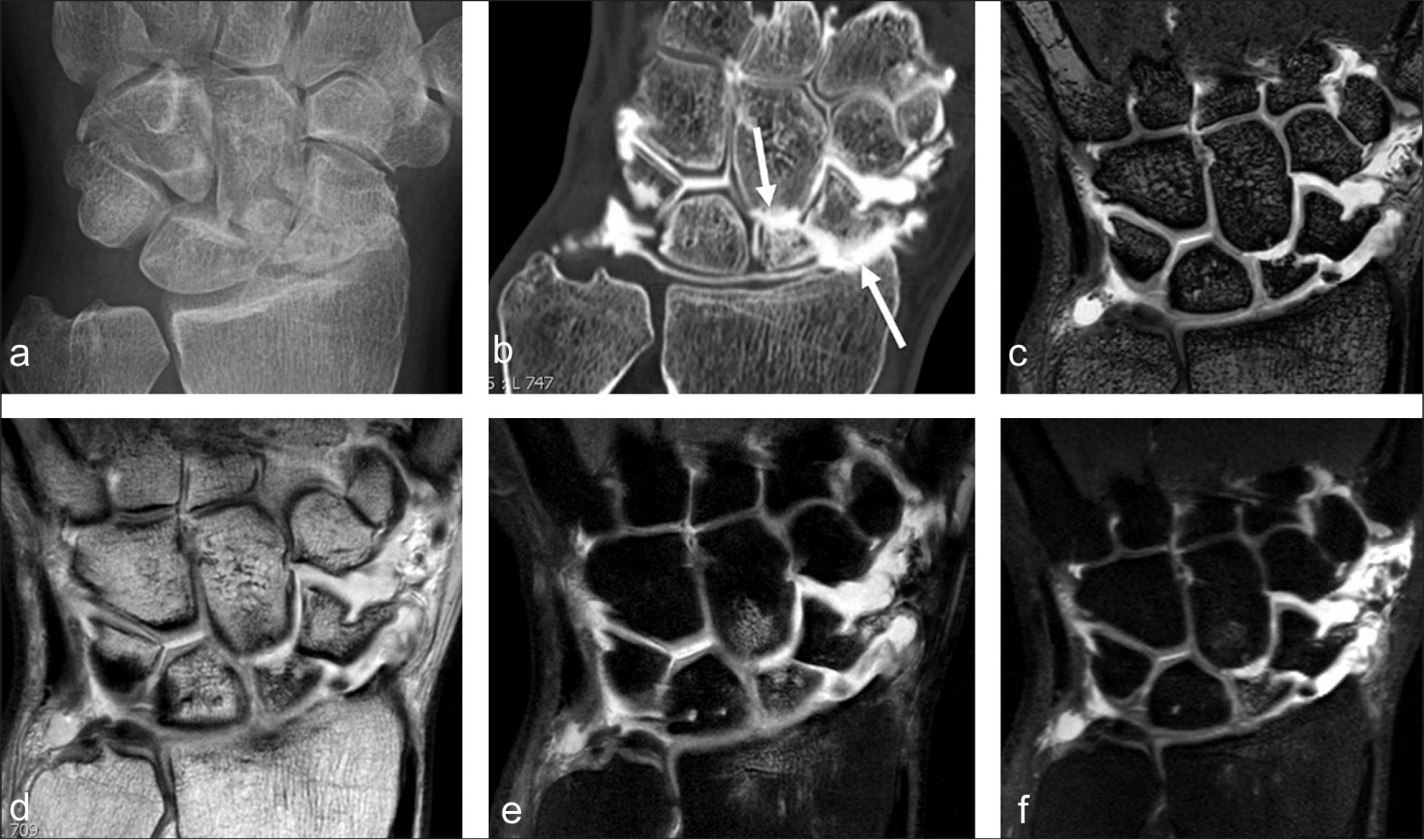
SNAC 2 wrist after scaphoid fracture with nonunion in a 41-year-old man. Secondary cartilage degeneration is first seen in the radioscaphoid compartment (b, arrow pointing up) and later in the scaphocapitate midcarpal joint space (b, arrow pointing down) on conventional radiograph and much better on CT-arthrography (b). MRI well demonstrates the nonunion (on 3D DESS with 0.4mm sections) and increased signal in the proximal scaphoid pole on 2D (e) and 3D (f) proton-density fat-saturated images, corresponding to edema and indicating that the separated fragment might be unstable (and painful). Subchondral bone marrow edema is also detected in the proximal pole of the capitate under the cartilage loss and in the distal radius (e).
